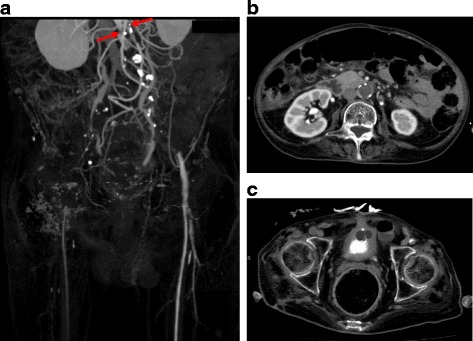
Preoperative computed tomography. The abdominal aorta is occluded below the renal arteries (a, *arrows*; b). The common, external, and internal iliac arteries are occluded bilaterally (a). The infrainguinal vessels were occluded on the right but patent on the left through the collateral vessels (a, c)
